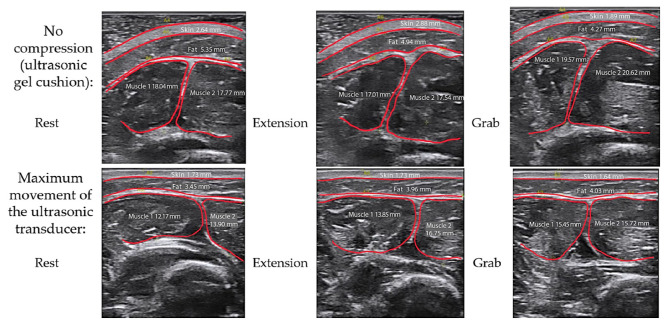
Ultrasound images of the internal anatomy of the forearm in the projection of the ulnar extensor muscle of the wrist and extensor digitorum muscles at rest, during extension of the hand and grasping without pressing and with the maximum pressing of the ultrasound sensor to the skin surface.
